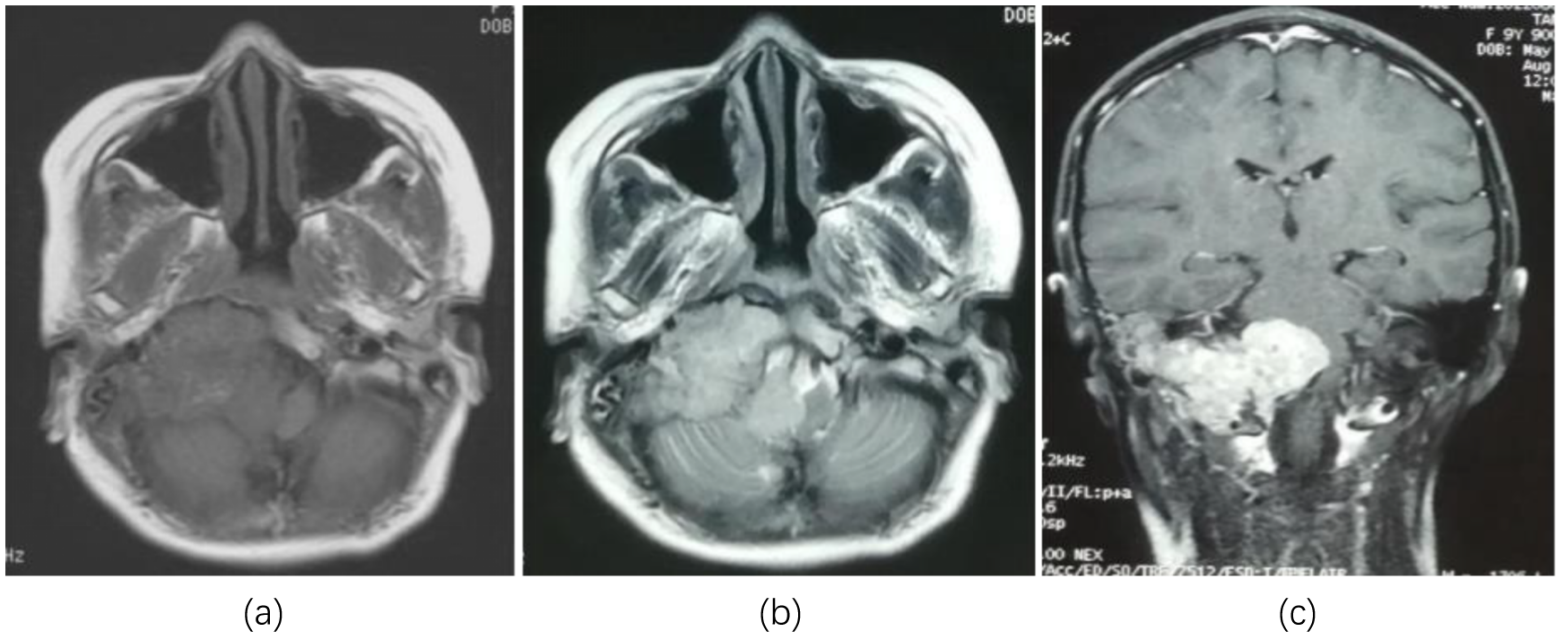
Head MRI showed lesions in the right and right anterior medulla oblongata, right slope area, right jugular foramen, right carotid sheath, and right parapharyngeal space occupying lesions. (a) Cross section T1WI scan shows a slightly equal length signal; (b) cross section T2WI scan showed uneven and slightly long signal; (c) Cross-sectional enhanced scanning showed obvious enhancement.
